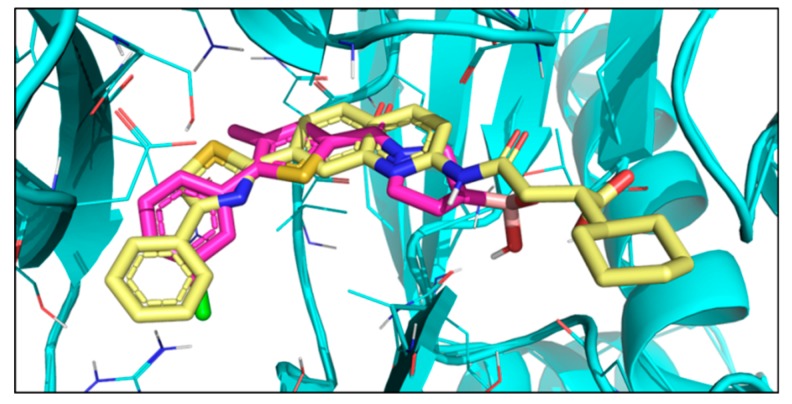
X-ray structure of Ro19 (yellow) (PDB code: 5M2B [16]) and docked pose of compound 1 (magenta).
